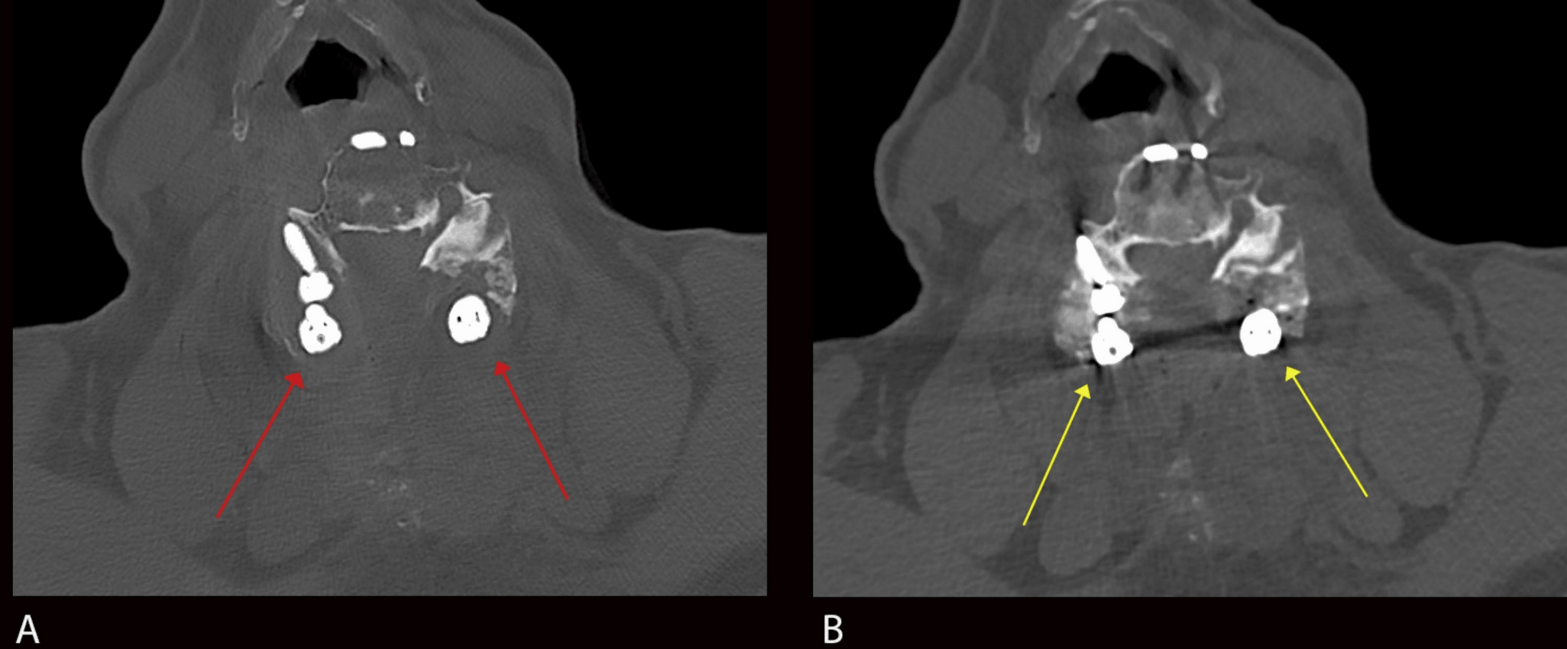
Axial image of the cervical spine with iMAR post-processing (A) and without iMAR post-processing (B) Figure 1A shows lucencies surrounding the pedicle screws at the C6 level (red arrows), mimicking findings associated with hardware loosening. These do not persist on axial CT images without iMAR post-processing (yellow arrows in Figure 1B) and the normal interface between the pedicle screws and osseous structures is shown. These findings are consistent with iMAR-related artifacts. iMAR: iterative metal artifact reduction
